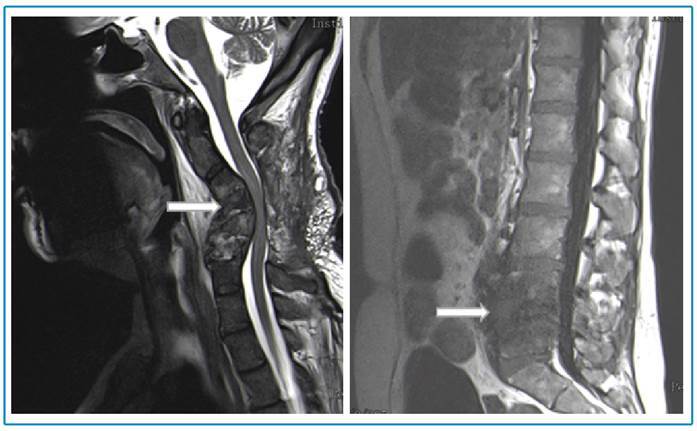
Magnetic resonance imaging reassessment after 7-week antibiotic course
